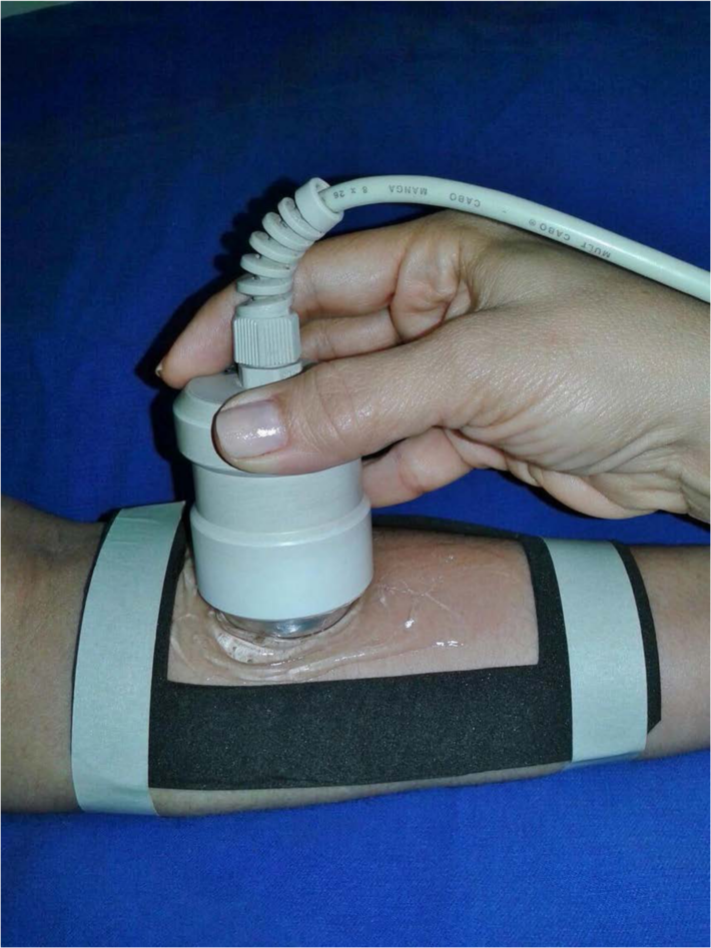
Application of therapeutic ultrasound in delimited area with neoprene template
